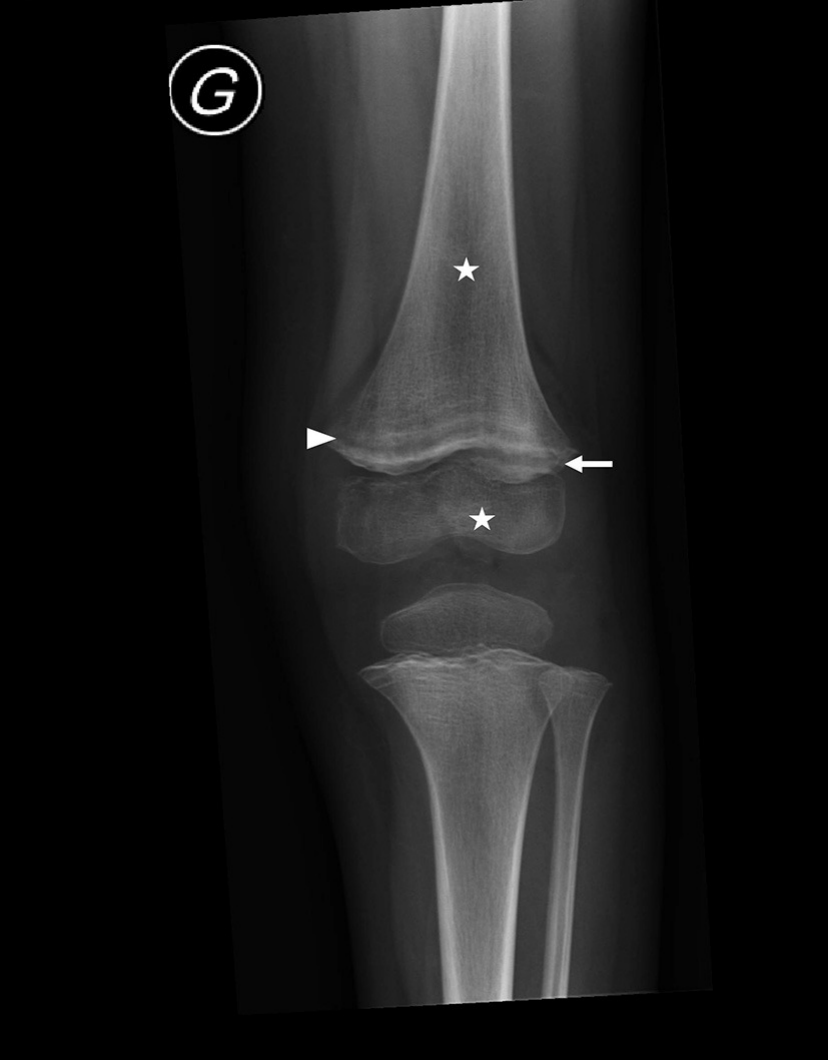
Left knee radiograph for case I, a 3-year-old boy, demonstrating multiple clear metaphyseal bands in the distal femur (white arrowhead), also defined as “Trummerfeld zone,” and marked white line corresponding to thickened zone of calcification, also named “white line of Frankel.” Irregular and enlarged metaphyseal margin (white arrow), as well as diffuse osteopenia (stars) are also noted.
